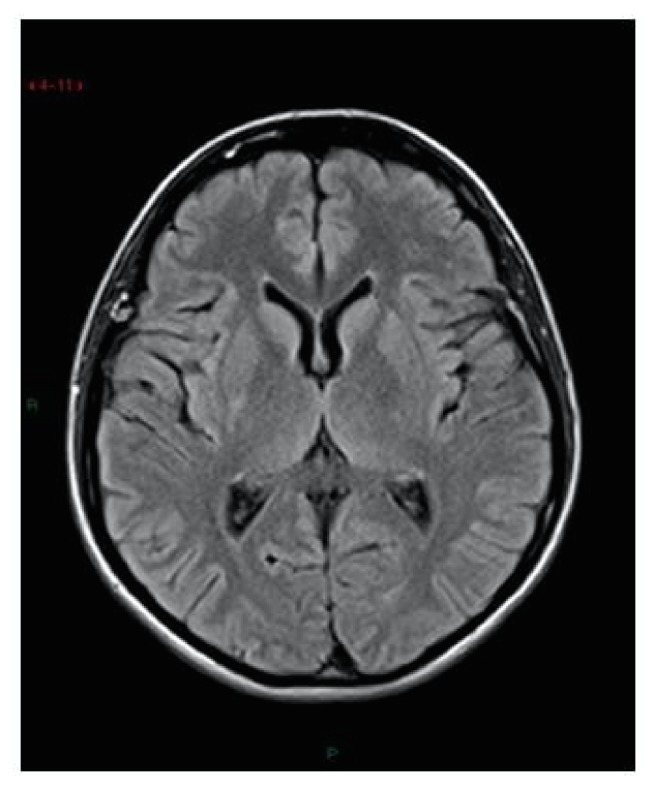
Magnetic resonance images performed 7 days after the first examination. There are no signs of foci of high signal intensity.
